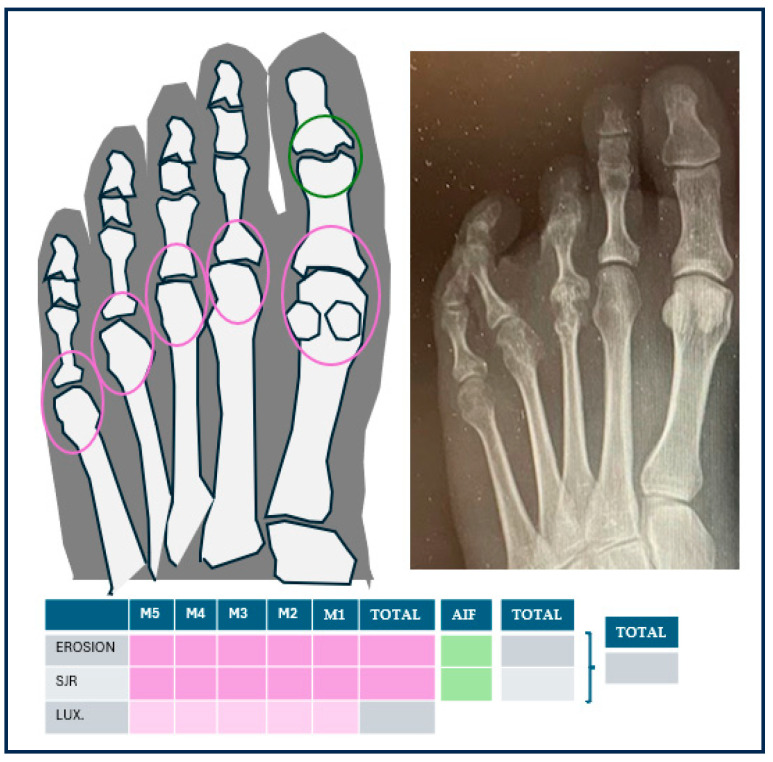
In the metatarsophalangeal joints (circled in pink) and the hallux interphalangeal joint (circled in green), the presence of erosions and decreased joint space were evaluated by summation. In addition, the presence of subluxation or dislocation of the metatarsophalangeal joints was indicated. On the right an example of a radiograph of a patient with rheumatoid arthritis.
